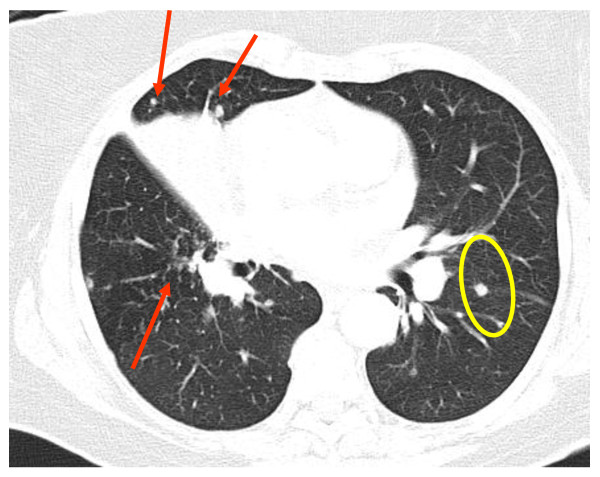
High-resolution computed tomography image of the chest at presentation (middle-lung zones). A mass-like lesion with nodules in the right (red arrows) and left (yellow circles) lung is shown.
